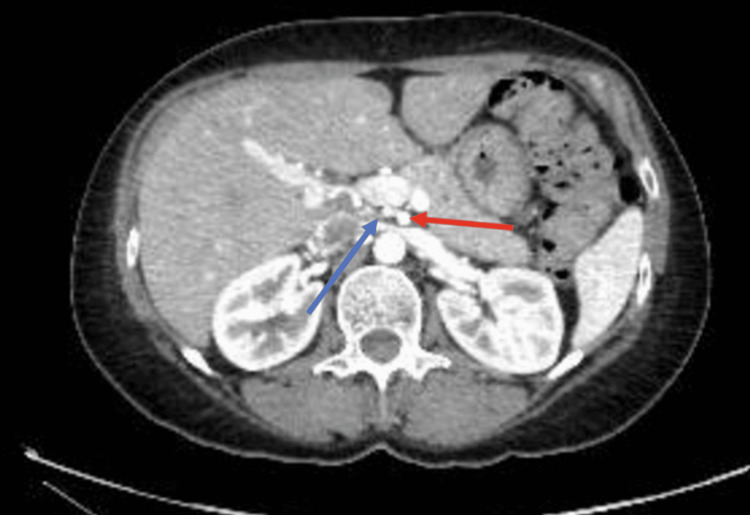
Axial CT demonstrating the right hepatic artery as it arises from the superior mesenteric artery The blue arrow denotes the proximal portion of the right hepatic artery. The red arrow denotes the superior mesenteric artery
